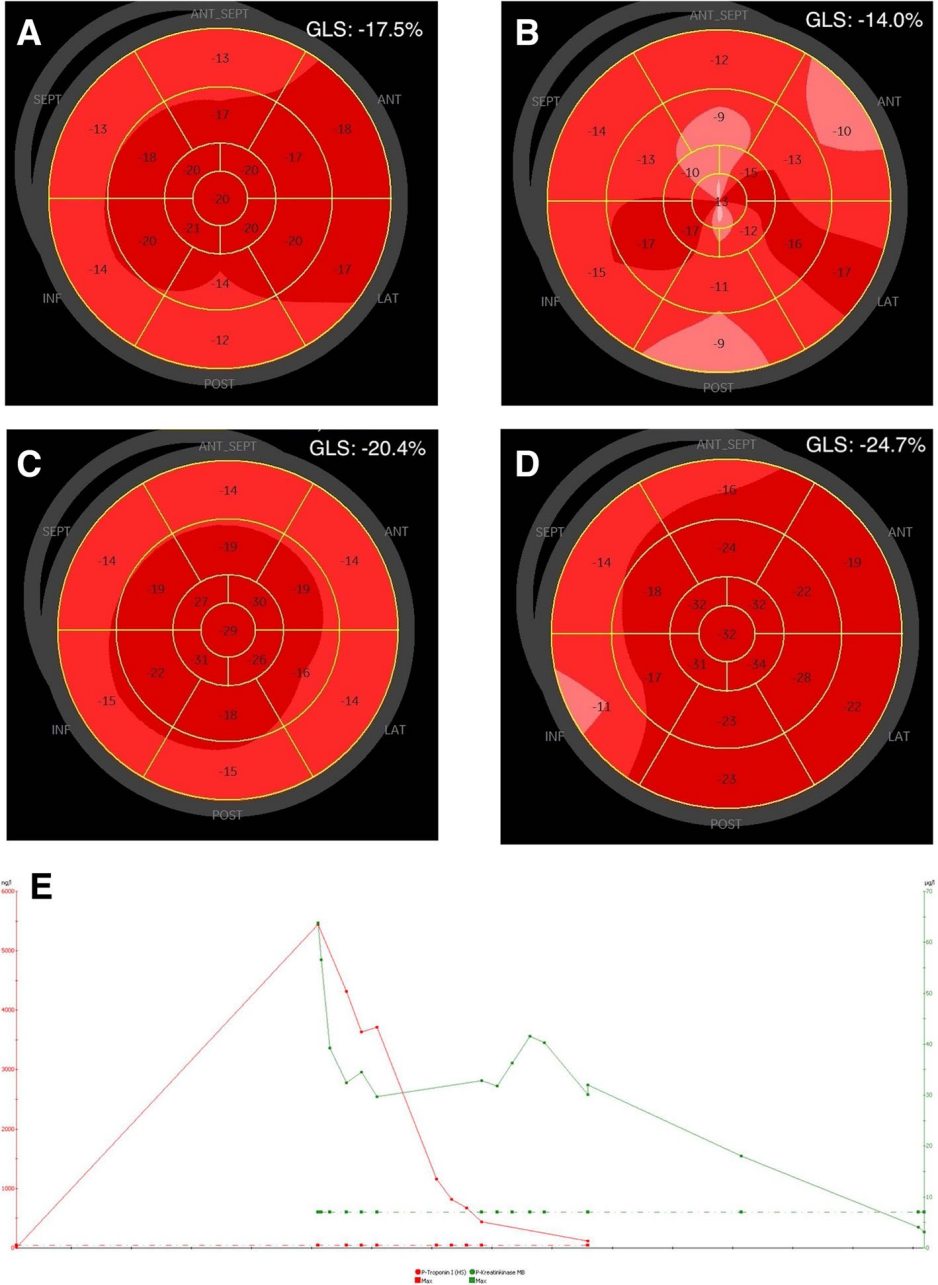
Two-dimensional transthoracic echocardiography (TTE) with global longitudinal strain (A-D) analysis and cardiac biomarkers (E). A TTE performed at the day of admission at the department of cardiology, at debut of symptoms, (cycle 2 day 12). B TTE performed on the day of peak of cardiac biomarkers, (cycle 2 day 14). C TTE performed approximately 1 year before admission. D TTE performed at the day of discharge, (cycle 2 day 21). E Cardiac biomarkers showing troponin peak. Troponin and CK-MB declined after initiation of high-dose steroid
